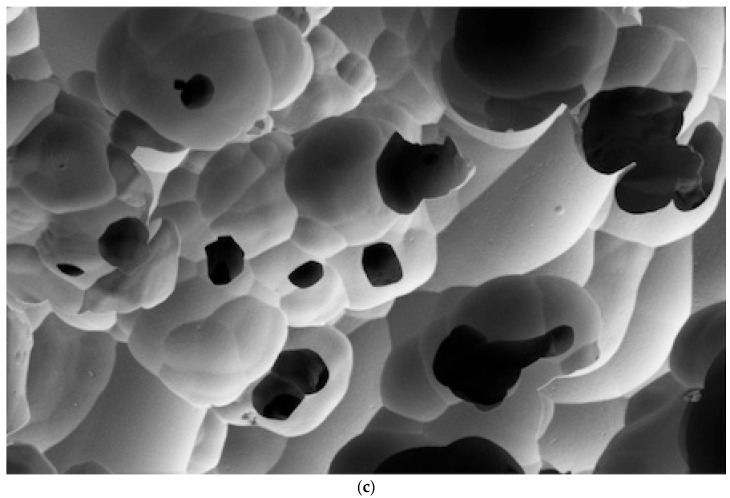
(a) The Variolink Try-in Paste cleaned with the Rinse and Dry method left a low amount of residual paste, covering less than 25% of the image frame. (b) The Variolink Try-in Paste cleaned with Rinse and Dry followed by an Ultrasonic Bath in Distilled Water showed a completely clean surface with no residual paste (Score: None). (c) The Variolink Try-in Paste cleaned with Rinse and Dry followed by an Ultrasonic Bath in Alcohol also showed a completely clean surface with no residual paste (Score: None).
